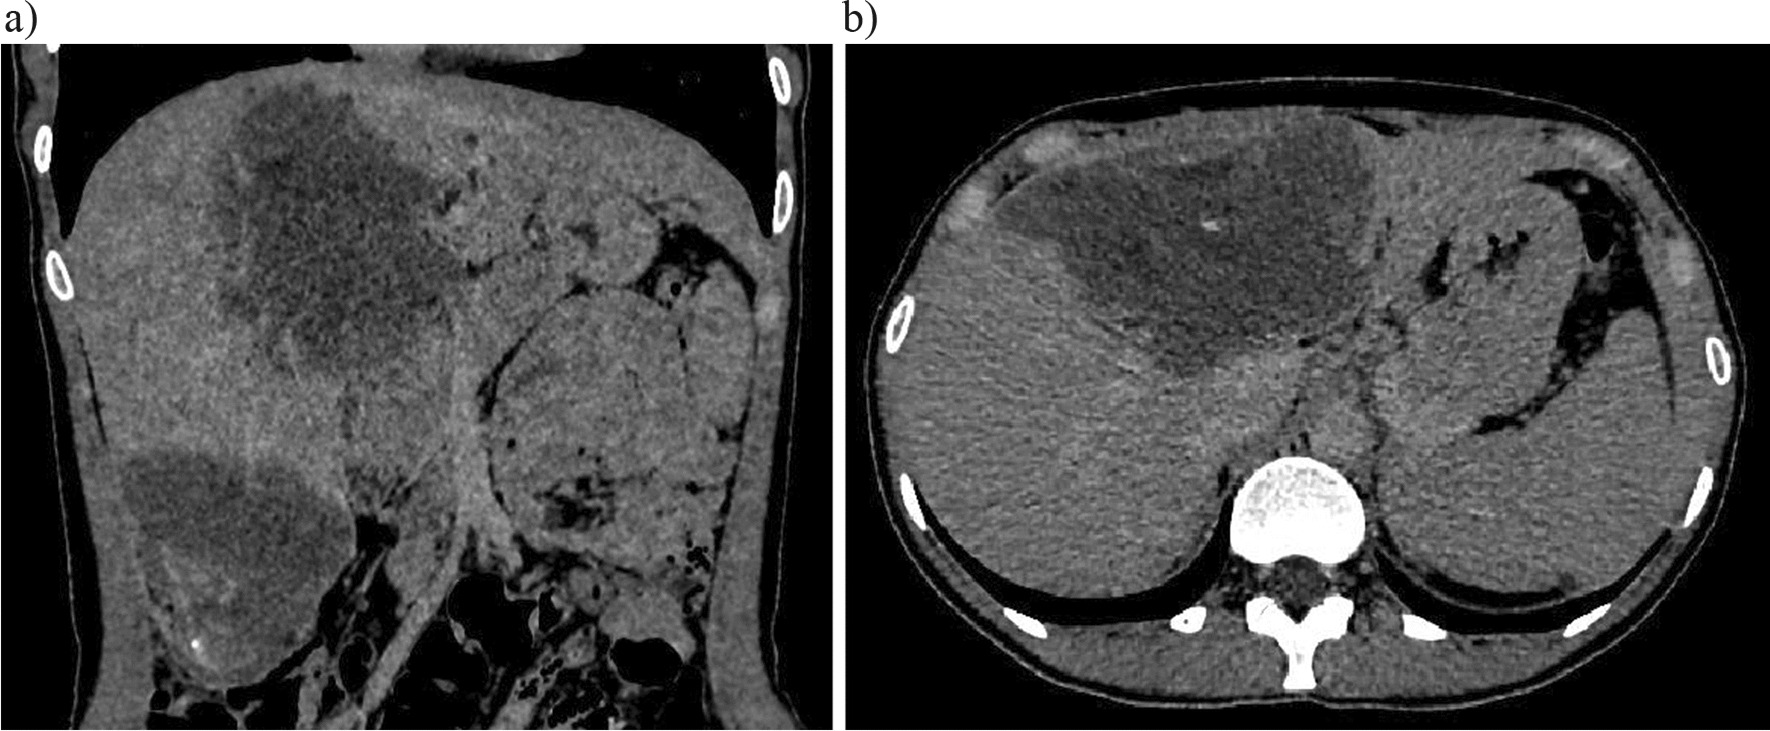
Coronal (a) and axial (b) CT image in the native phase demonstrates tumor masses in IV, VIII and partially V, VI segments. Uneven fuzzy contours, signs of infiltrative growth into the liver parenchyma, cystic-solid structure with + 10 to + 40 HU, single calcified inclusions (+ 145HU), invasion into the Glisson’s capsule, dilatation of the bile ducts along the tumor periphery
